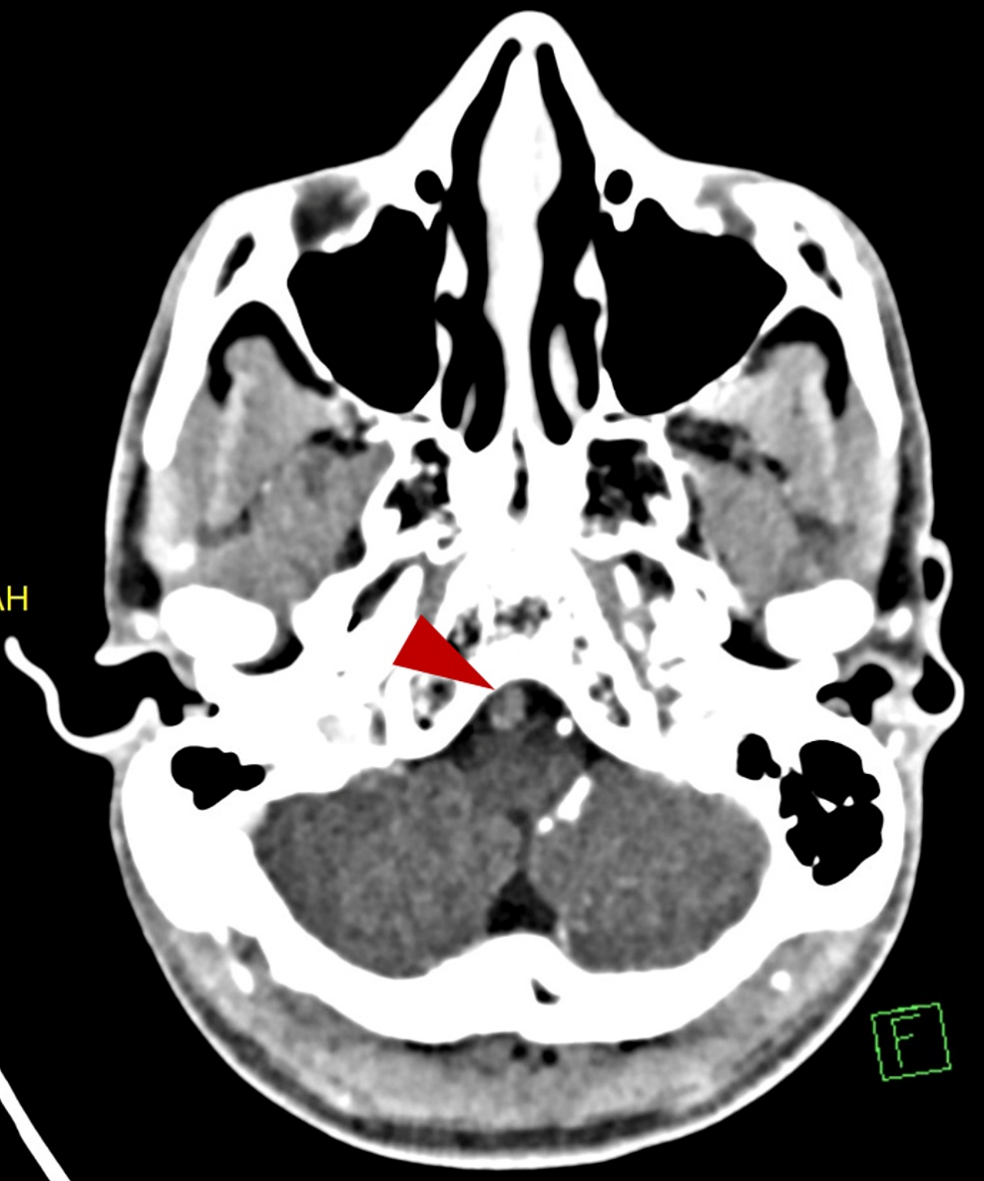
CT angiography. Arrowhead showing vertebral artery dissection with a thrombosed aneurysm.
